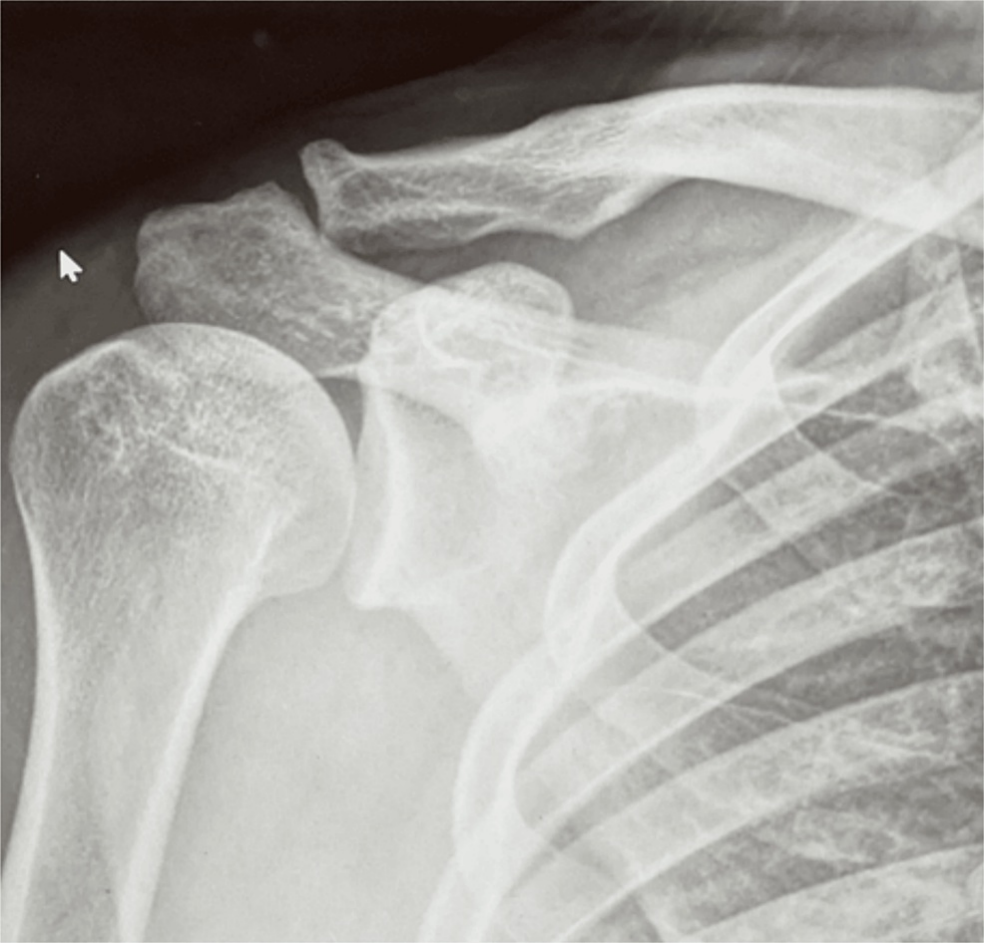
Right shoulder X-ray. No fracture or dislocation is noticed.
